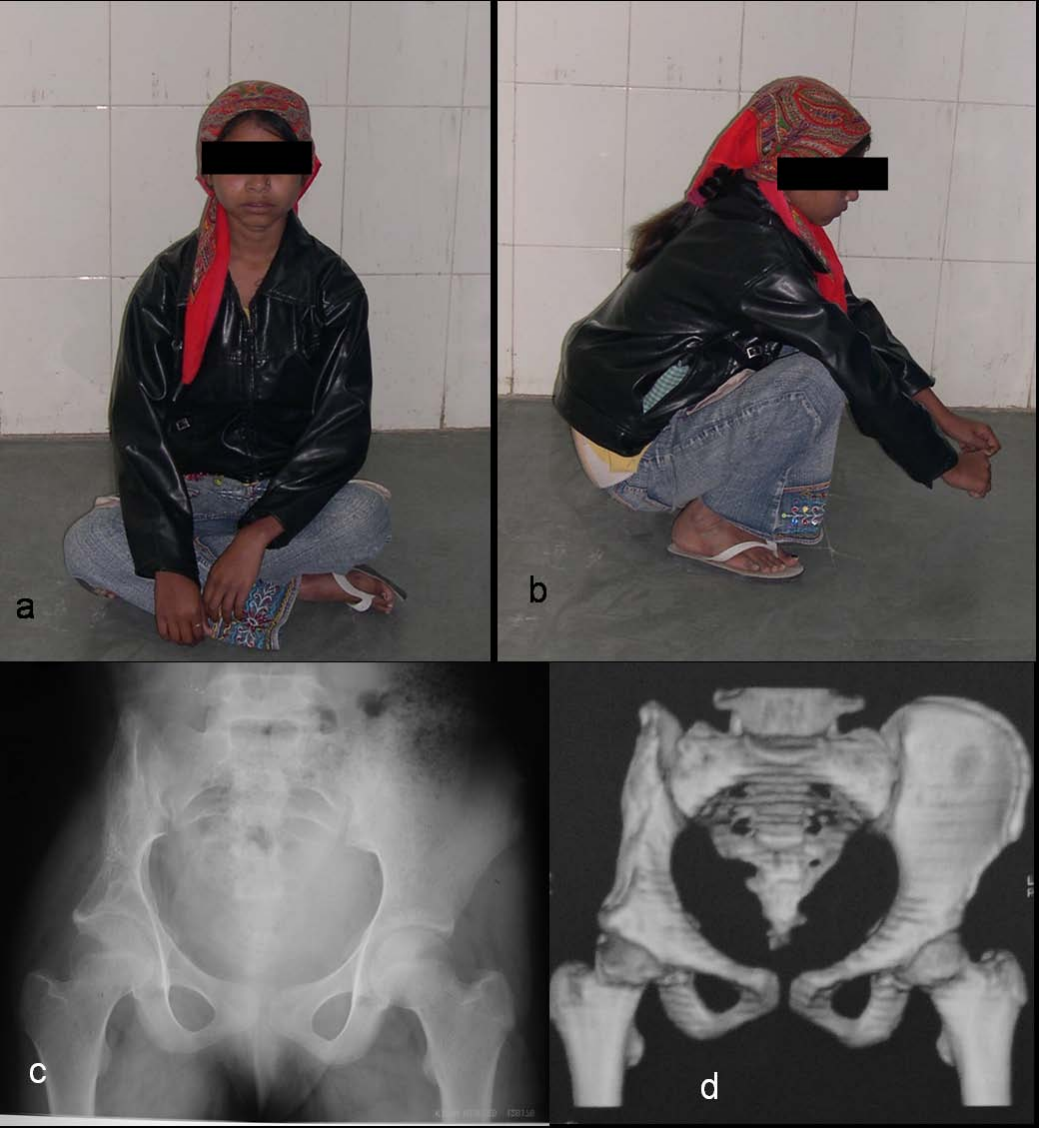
a, b) Follow up 18 months: comfortable cross legged sitting and squatting. c) Plain radiographs and d) CT showing good remodeling and no involvement of the hip joint.
